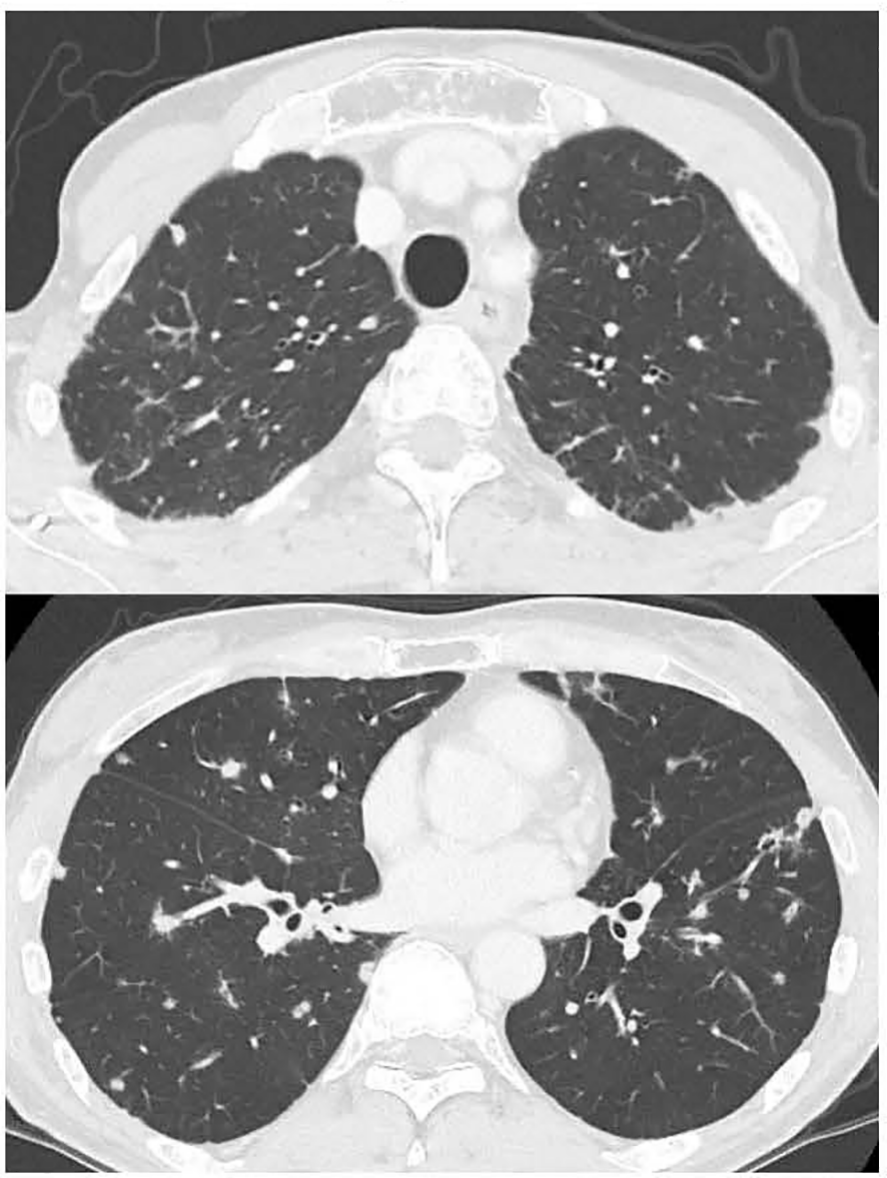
Chest CT showing multiple lung involvements at initial diagnosis.
